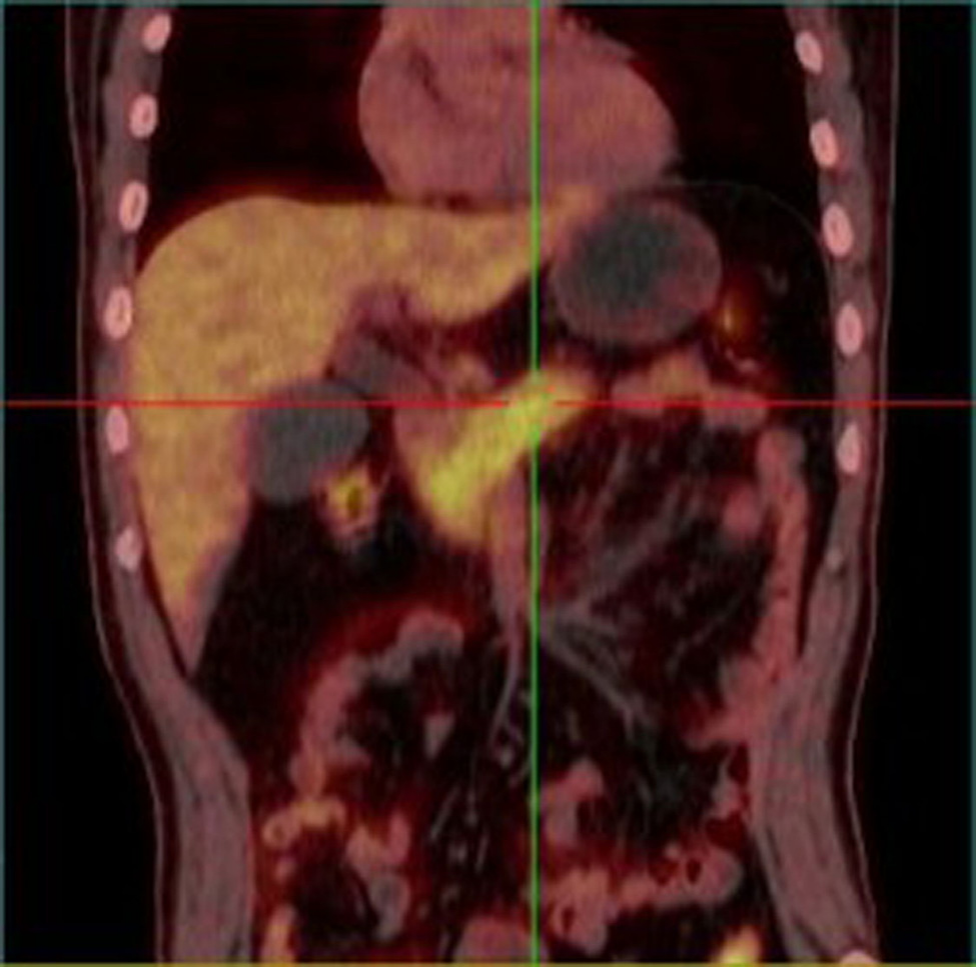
PET/CT reveals swelling of the pancreas with diffuse increase in glucose metabolism, especially in the head and neck region.
